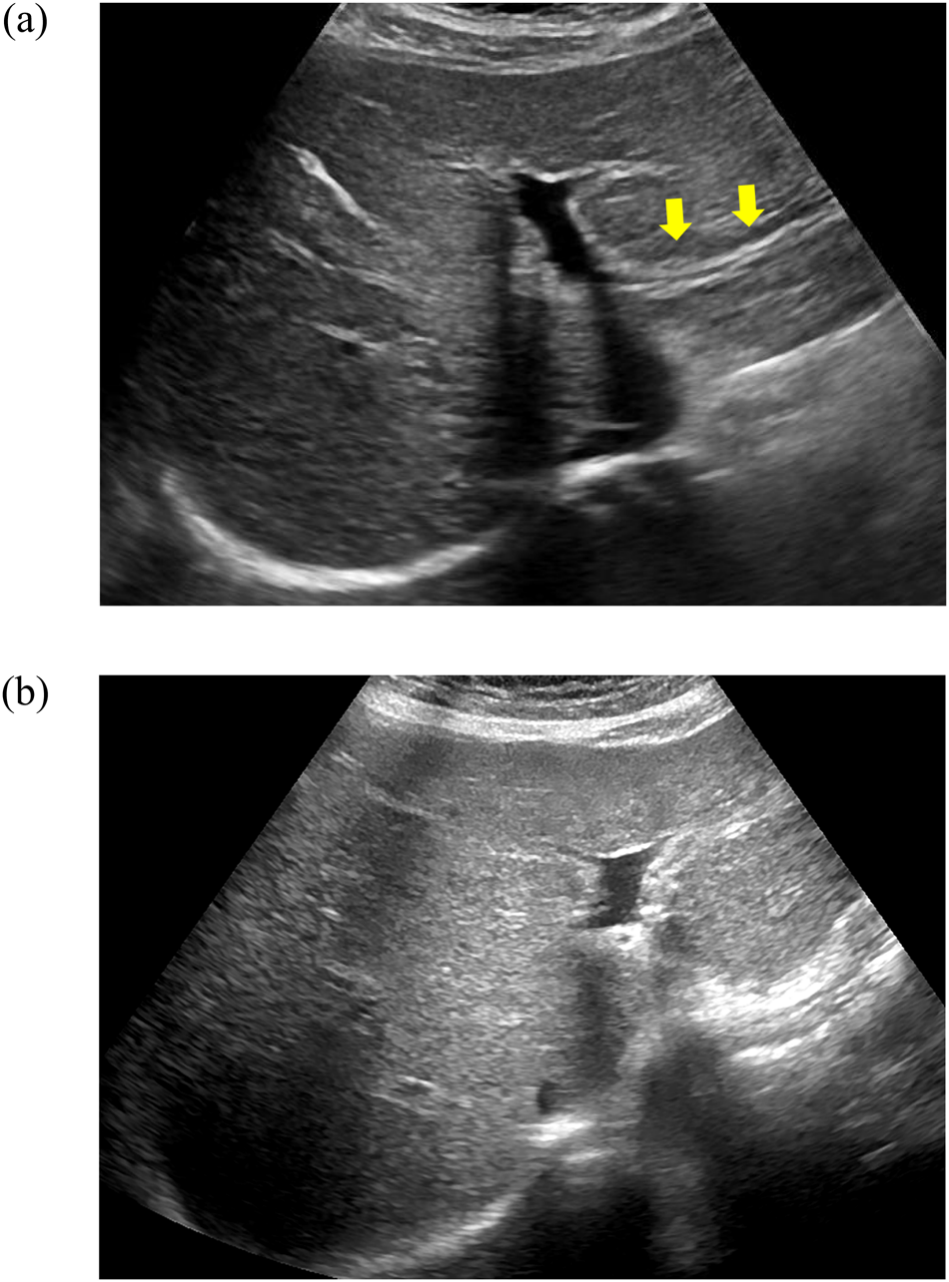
With and without PHB around the portal vein on ultrasound. a Hypoechoic band can be seen around the portal vein. b Hypoechoic band can not be seen around the portal vein. PHB, periportal hypoechoic band
